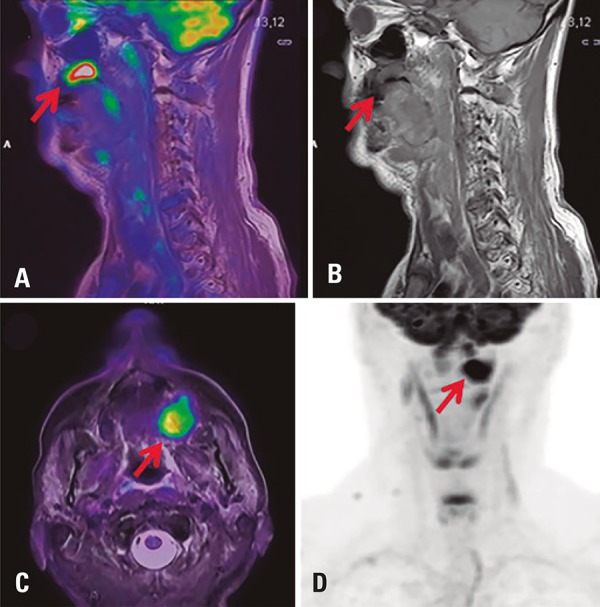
Head-and-neck 18F-FDG PET/MRI images showing hypermetabolic uptake in the left anterior portion of the gum line, extending to adjacent structures. (A) fused PET/MRI image, sagittal T1; (B) sagittal T1; (C) fused PET/MRI image, axial T2 fat saturated; (D) PET, maximum intensity projection image (arrows)
